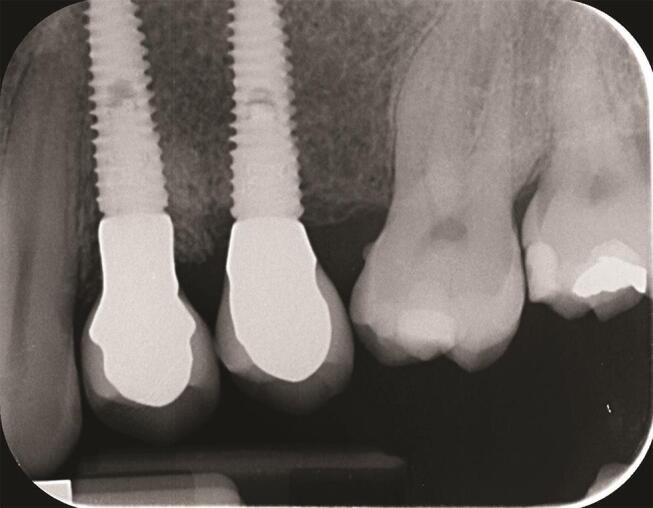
Periapical radiograph at the definitive prosthesis delivery.
